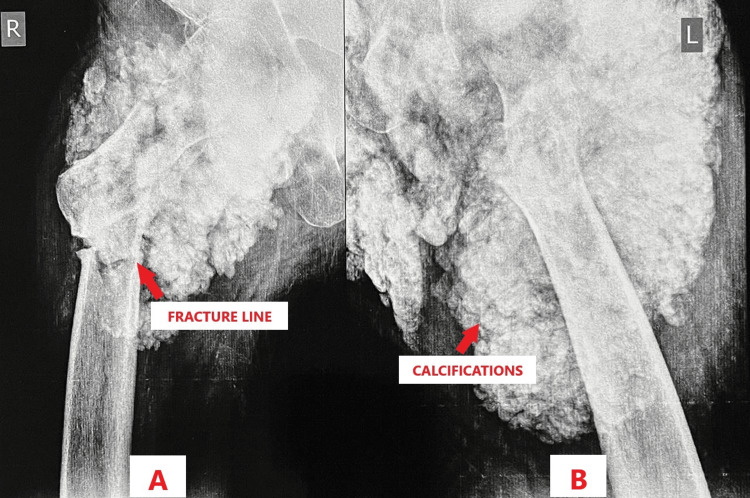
Radiograph of cross-table lateral views of the right and left hip. 2A: Right cross-table lateral view showing the fracture line. 2B: Left cross-table lateral view showing peri-articular calcific depositions.
